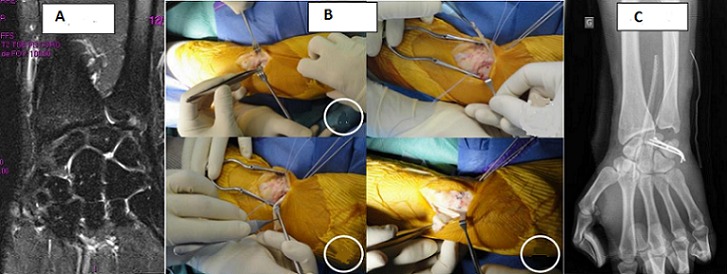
A) MRI of the wirst showing TFCC lesion; B) operative technique of lunotriquetral instability repair; C) three lunotriquetral K wires protecting the ligamentoplasty
